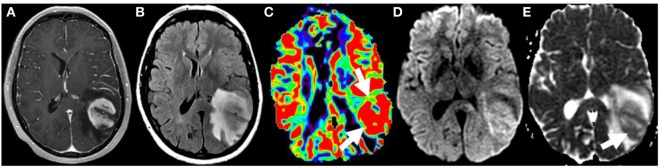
Glioblastoma on initial presentation. T1W postgadolinium (A) and T2W FLAIR (B) magnetic resonance sequences demonstrate a large intra-axial heterogeneously enhancing left parietal mass with extensive surrounding edema and/or infiltrative neoplasm. Elevated relative cerebral blood volume (arrows) is noted on the dynamic susceptibility contrast perfusion map (C) compatible with increased vascular density. The diffusion-weighted imaging (D) and the corresponding apparent diffusion coefficient map (E) demonstrate heterogeneous restricted water motion (arrow) within this mass most compatible with hypercellularity. No copyright permissions were required to use these images.
